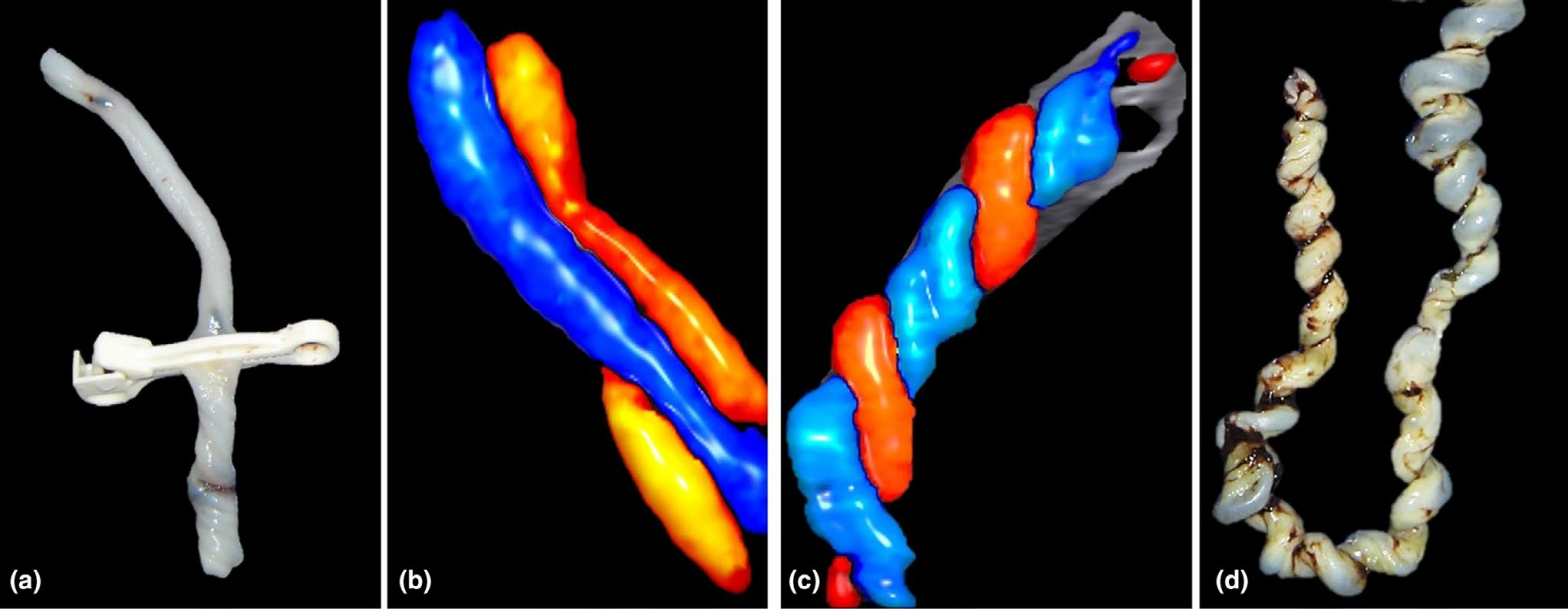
Abnormal coiling. (a, b) Macroscopic and ultrasound appearance of hypocoiled or (c, d) hypercoiled umbilical cord. Although these umbilical cords' abnormal cooling might affect cord blood flow and fetal hemodynamics, the heterogeneity of existing studies prevents the establishment of definitive causal links or evidence‐based preventive strategies.
